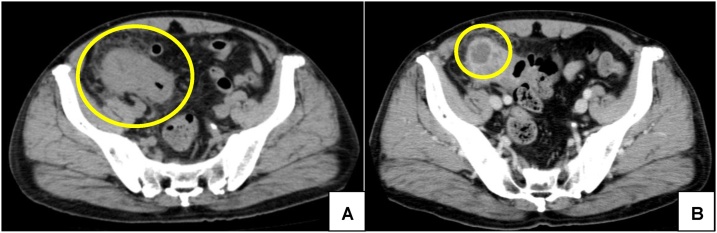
Enhanced abdominal computed tomography scan. A. 45 mm × 55 mm irregular ileocecal wall thickness with enhancement. B. Low density mass such as an abscess caudal of the tumor.
